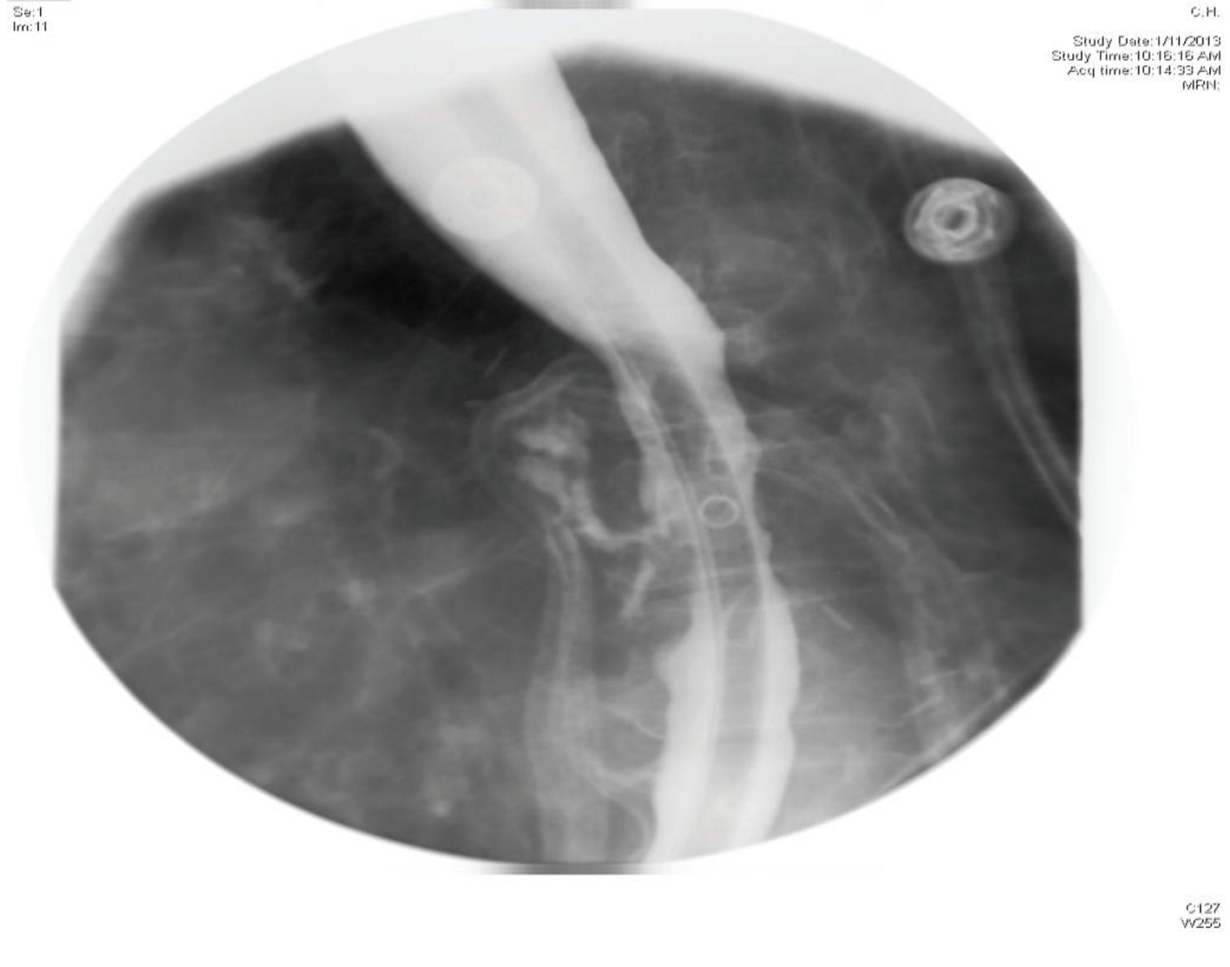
X-ray esophagus with gastrografin showing sinus tracts extending from anterior aspect of esophagus.
